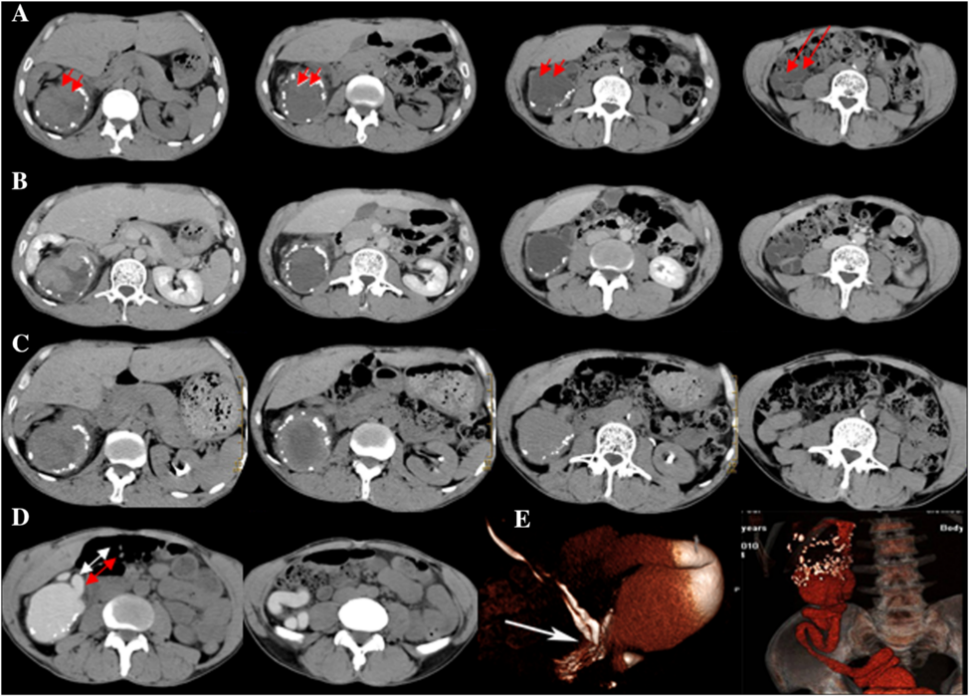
Case 1. A 56-year-old male patient with right side duplex kidney. Unenhanced supine axial CT (a) shows a solitary round, iso-dense, soft-tissue mass with a clear boundary (*short arrow*). The irregularly annular calcified shadow around the mass was equivalent to multiple annular low-density shadows in the ileocecal junction (*long arrow*). The mass was not clearly intensified after contrast enhancement (b) and one-hour delayed (c) CTU scanning. Interestingly, it was obviously strengthened after 18-h delay (d), confirming duplex kidney. Furthermore, we found a band of high-density shadows that was confirmed to be duplicated ureters (*double-headed arrow*). Reformatted 3D CTU after segmentation of bone structures also showed the entire course of the dilated ureters (e)
